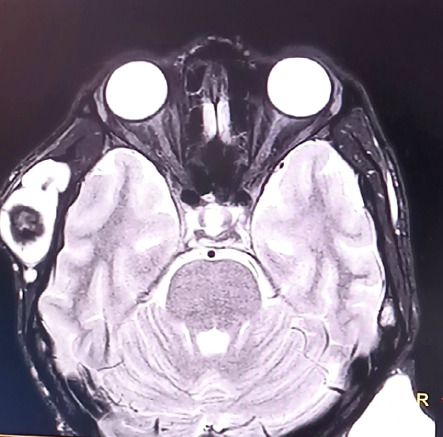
An axial T2-weighted MRI of the brain shows both the orbits with no features of surface occupying lesions.
